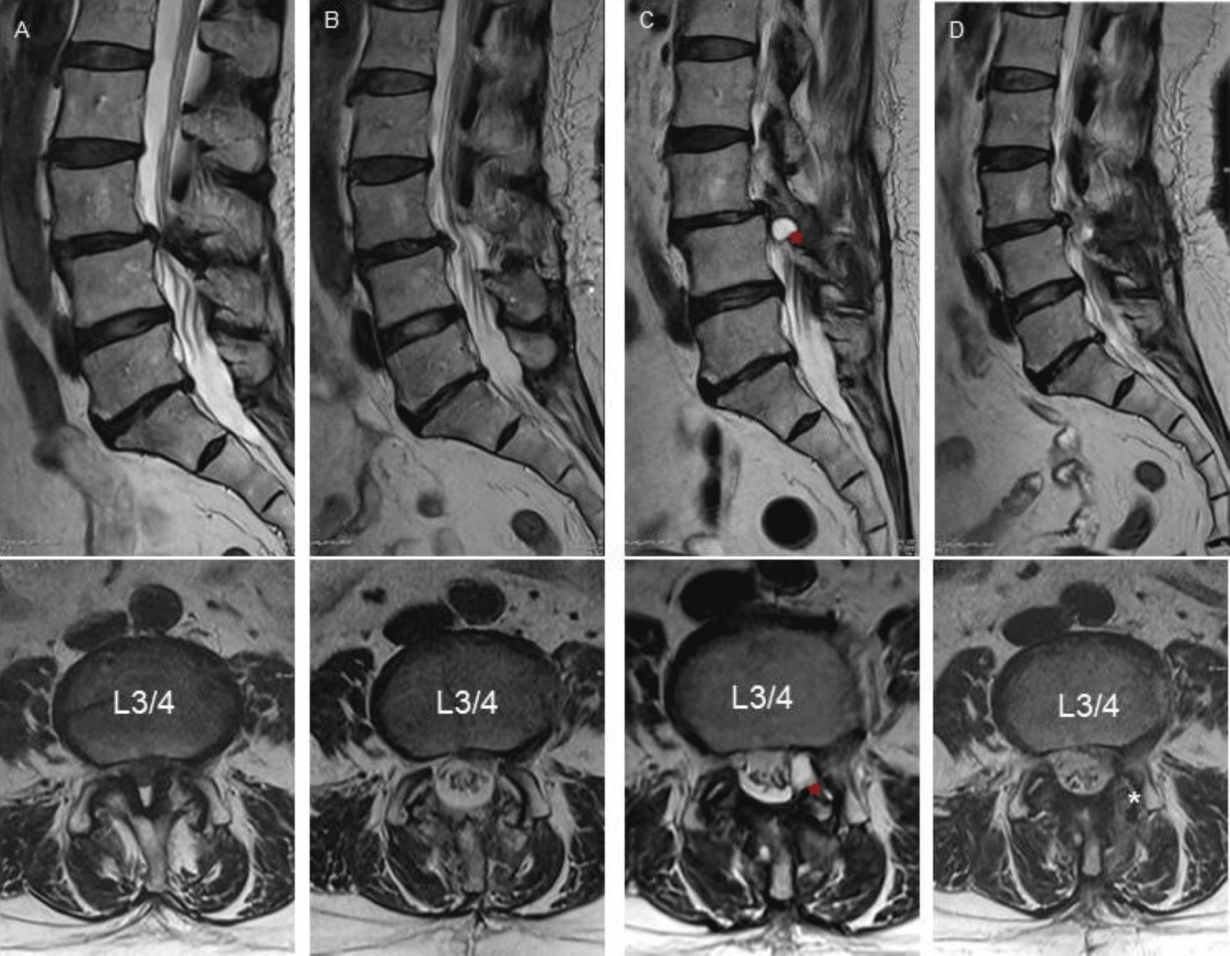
Case 1 MRI Progression Timeline Sagittal and axial T2-weighted MRI. Preoperative images (L3-4 LSS + DSL Grade 1) (A). Post-ILP decompression state (B). FC (red asterisk *) development and lateral recess stenosis (C). Post-FC resection and lateral recess decompression state (D). LSS: lumbar spinal stenosis, DSL: degenerative spondylolisthesis, ILP: interspinous ligamentoplasty, FC: facet cyst.
